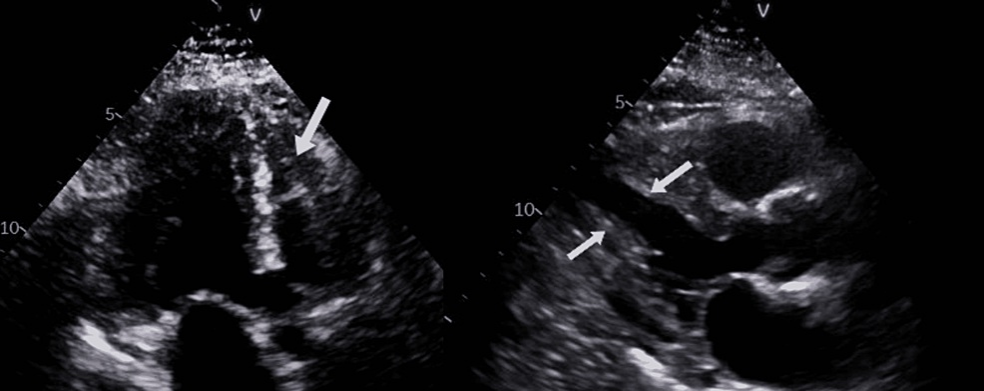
Echocardiogram findings (white arrows) suggestive of mildly increased left ventricular wall thickness with preserved left ventricular ejection fraction (LVEF) and a normal right ventricular systolic function.
